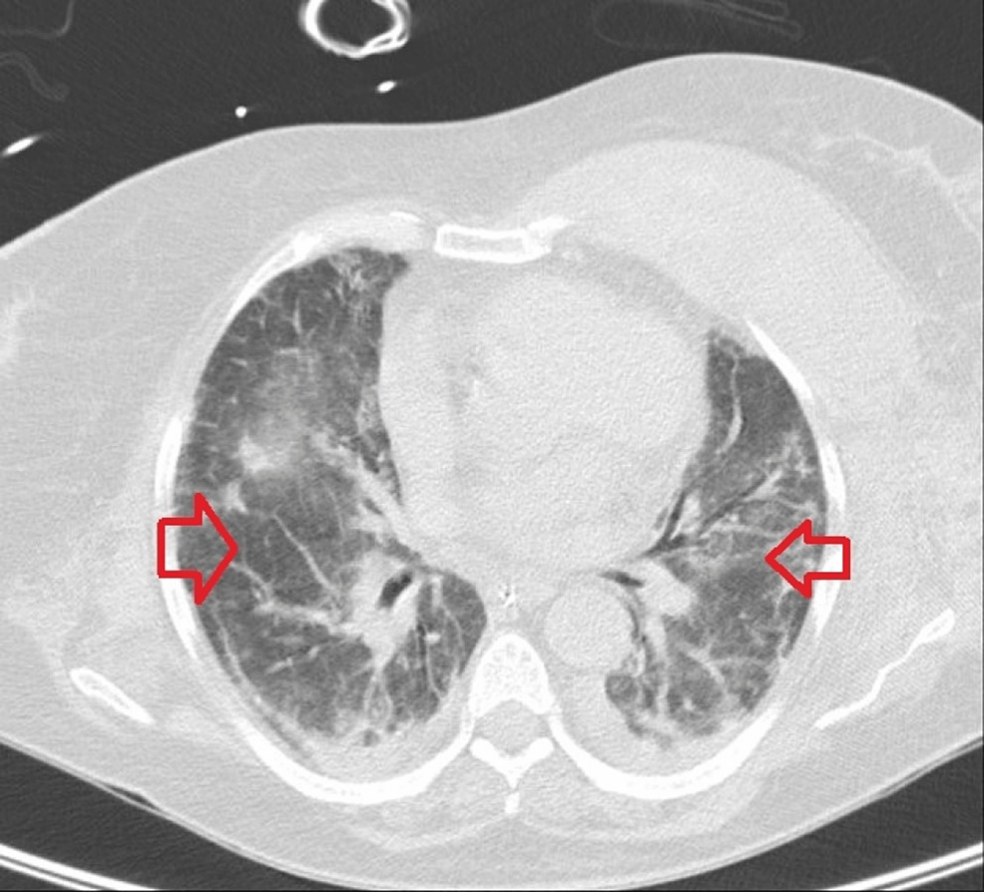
CT scan (axial view) corresponding to the sixth day of methylprednisolone administration The image shows an improvement of pulmonary condensation (red arrow)
